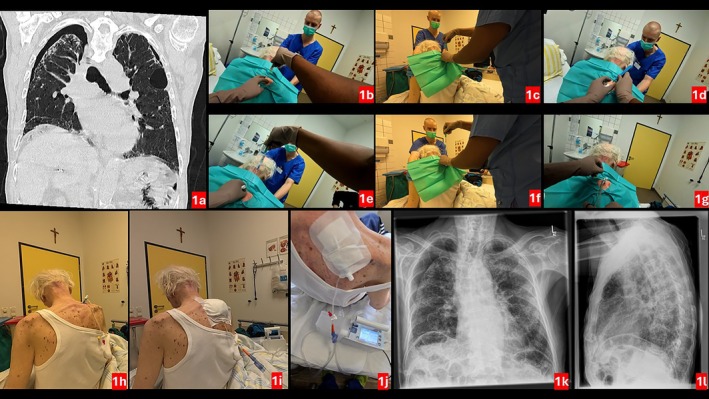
(a) Initial CT‐scan showing apical pneumothorax. (b, c) Local anaesthetic infiltration. (d) Skin incision before chest tube insertion. (e, f) Insertion of chest drain with trocar. (g) Chest drain suture placement. (h–j) Attachment to portable electronic chest drainage system. (k, l) Chest x‐ray showing apical placement of chest tube.
